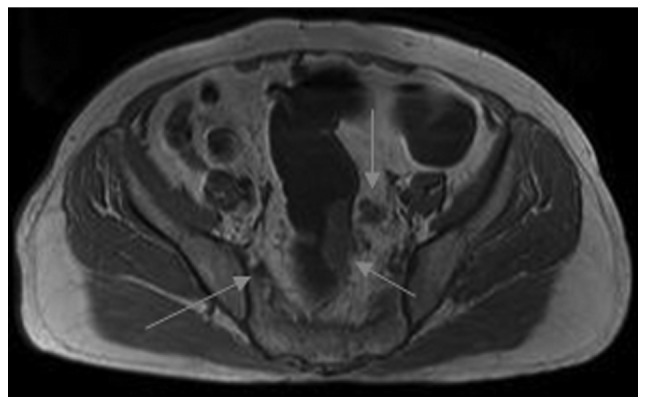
T1-weighted image without fat suppression showed tumour invasion of the surrounding fat tissue and two lymph nodes showed the same signal intensity at the left and right side of the intestinal walls with irregular shapes.
